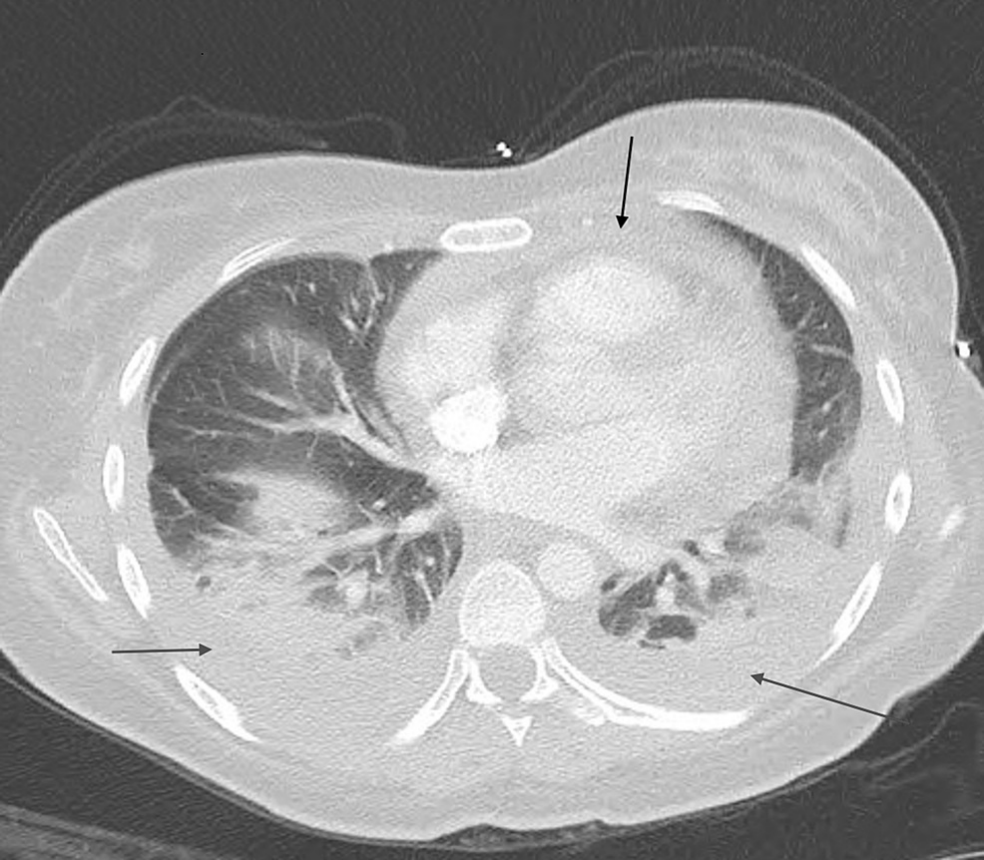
CTA with and without contrast demonstrating no pulmonary embolism, pleural effusions with bilateral basilar atelectasis or consolidation (demonstrated by grey arrows), stable pericardial effusion (demonstrated by black arrow).
